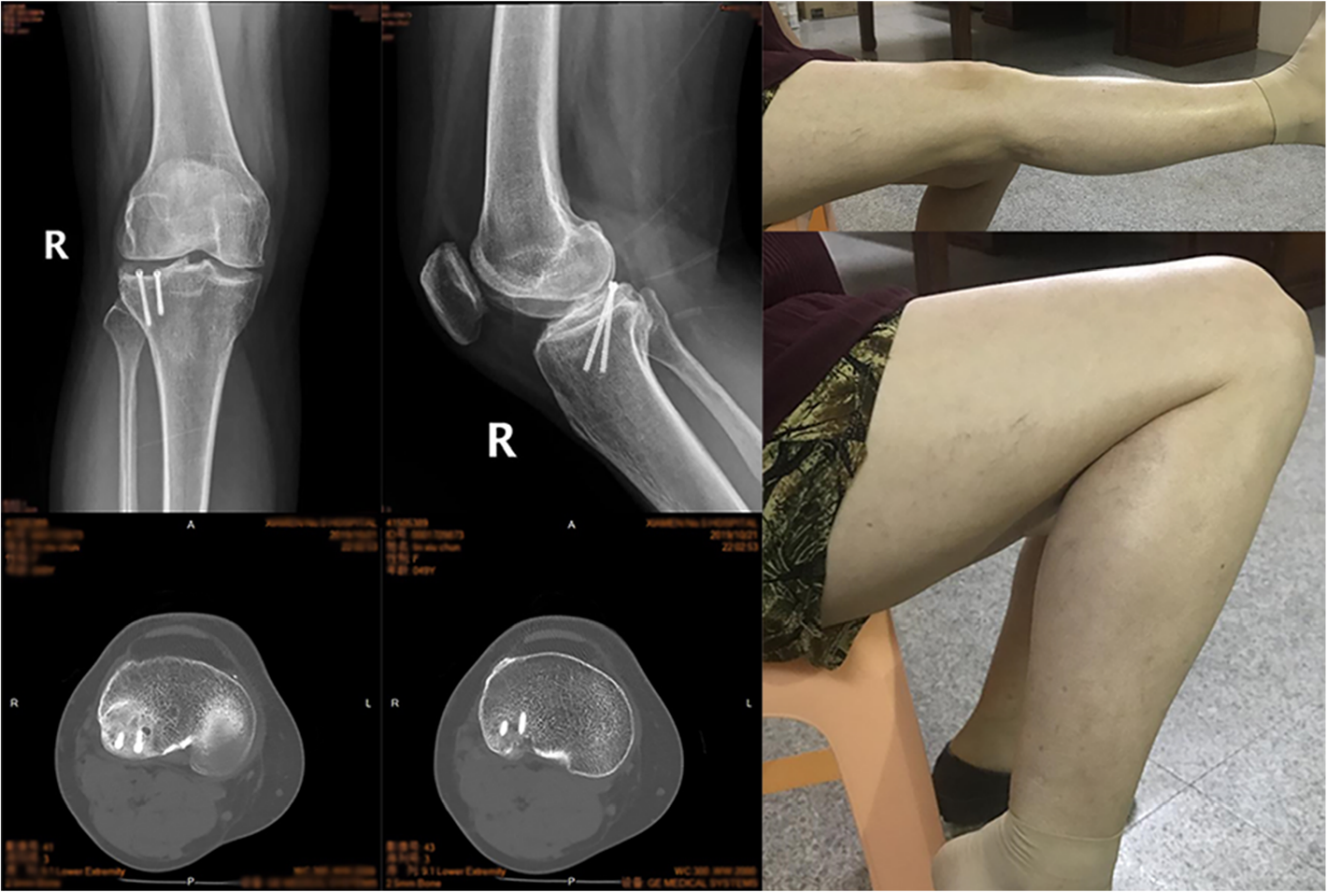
At the final fourth-year follow-up, X-ray and CT showed the fracture was healed without avascular necrosis, and a good ROM (0-145°) with limited discomfort was achieved
